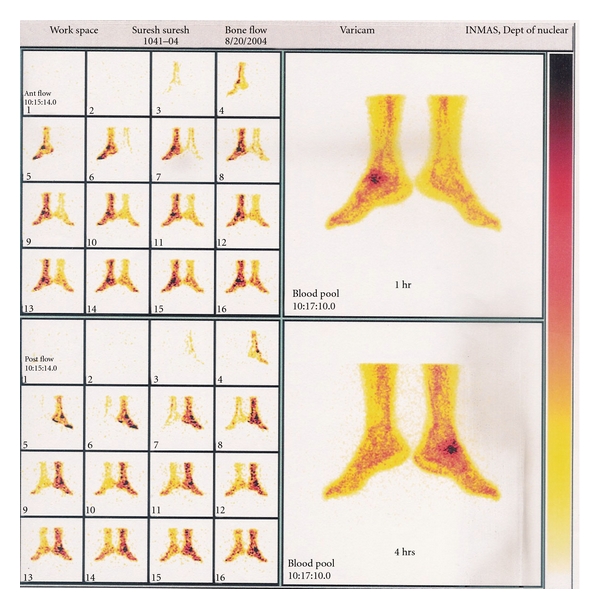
SPECT and scintigrams of patient, showing preferential localization of radiotracer in the ankle lesion after 1 hr and 4 hours postinjection of 370 MBq of ^99m^Tc-EMB.
